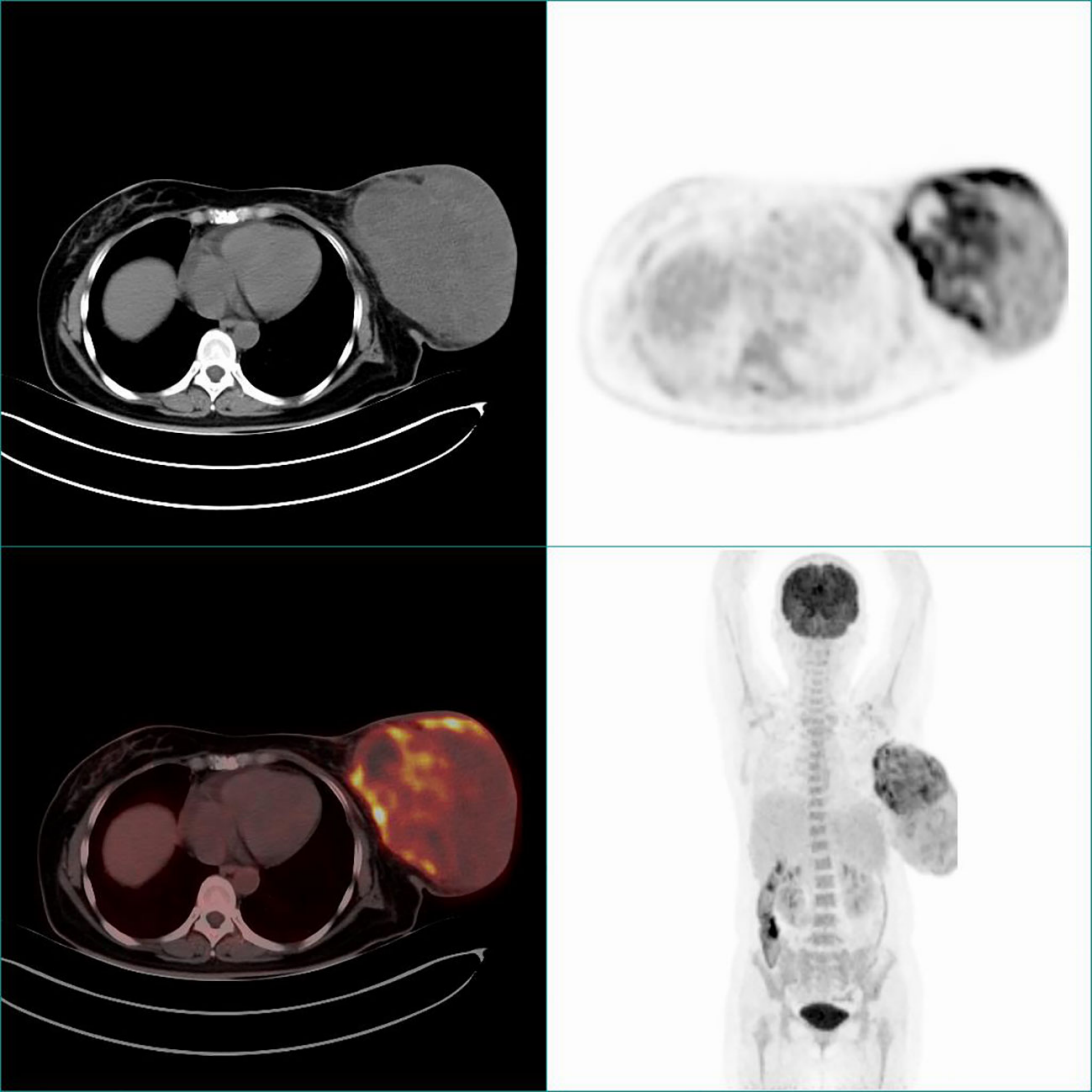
Preoperative PET-CT images.
